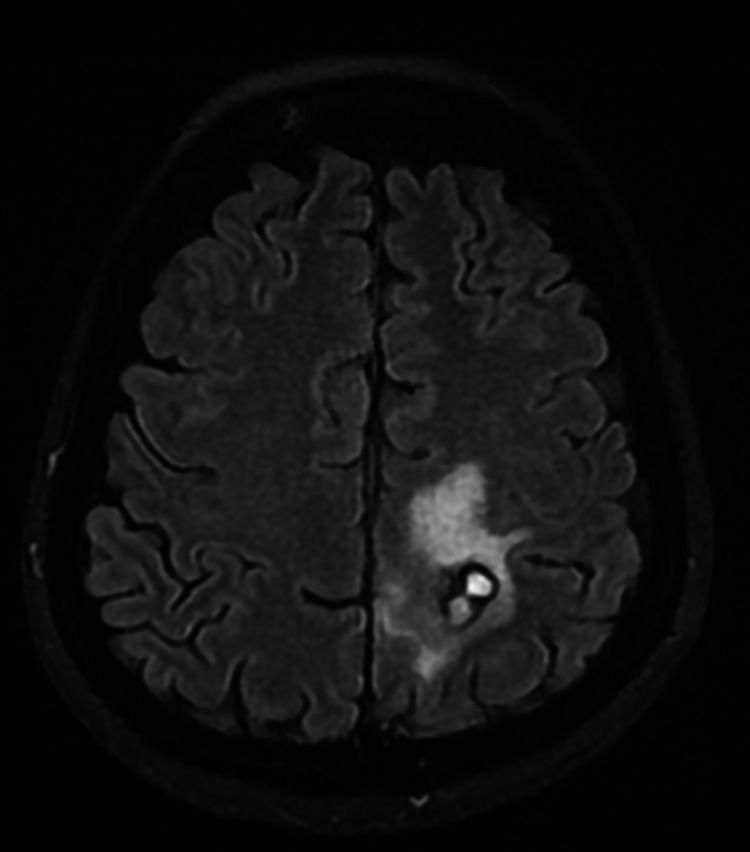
CT brain T2 axial view shows interval regression in the size of the known left partial and posterior frontal abscesses with improvement of the surrounding vasogenic edema.
